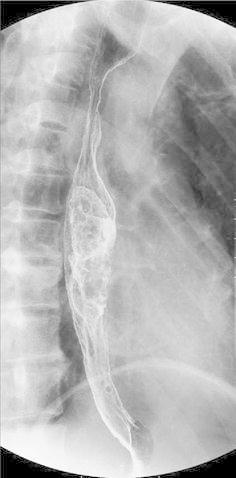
Upper gastrointestinal contrast radiography shows a pedunculated tumor in the mid-esophagus
